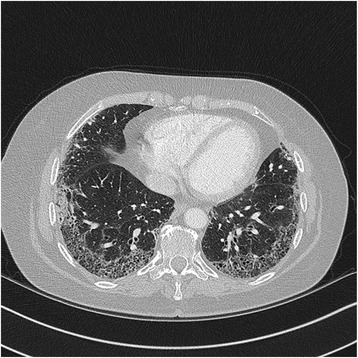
High-resolution computed tomography scan showing extensive peripheral, subpleural pulmonary fibrosis, and early honeycombing
